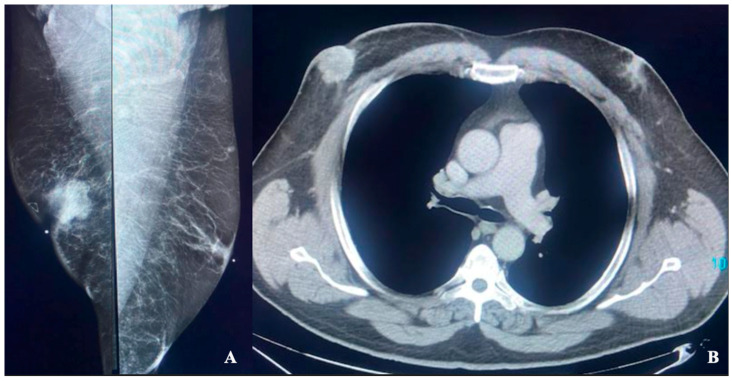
(A,B) Mass in the right breast and gynecomastia in a patient presenting with a palpable lump in the right breast. (A) Bilateral MLO view demonstrates a right retroareolar mass with nipple retraction and skin involvement at the site of the triangular skin marker denoting the location of the palpable lump. Biopsy revealed invasive ductal carcinoma. Subareolar linear opacities are seen on the left consistent with dendritic gynecomastia. (B) Axial CT of the chest demonstrates a right retroareolar mass, which was demonstrated to be breast cancer at biopsy. On the left, retroareolar breast tissue measures over 2 cm, consistent with gynecomastia.
